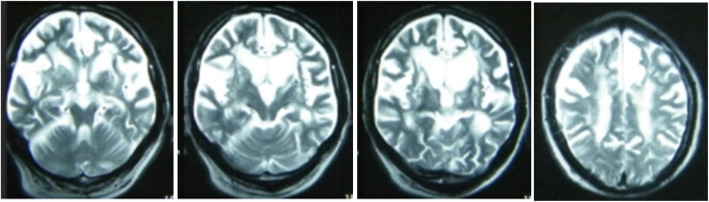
Patient’s brain MRI images Severe atrophy in the bilateral temporal and frontal lobes, mild atrophy in parietal lobes, as well as lacunar infarction in bilateral radiation coronal, basal ganglia, left thalamus and brainstem. Mild white matter hyperintensities (WMHs) can be found on both sides lateral ventricle
